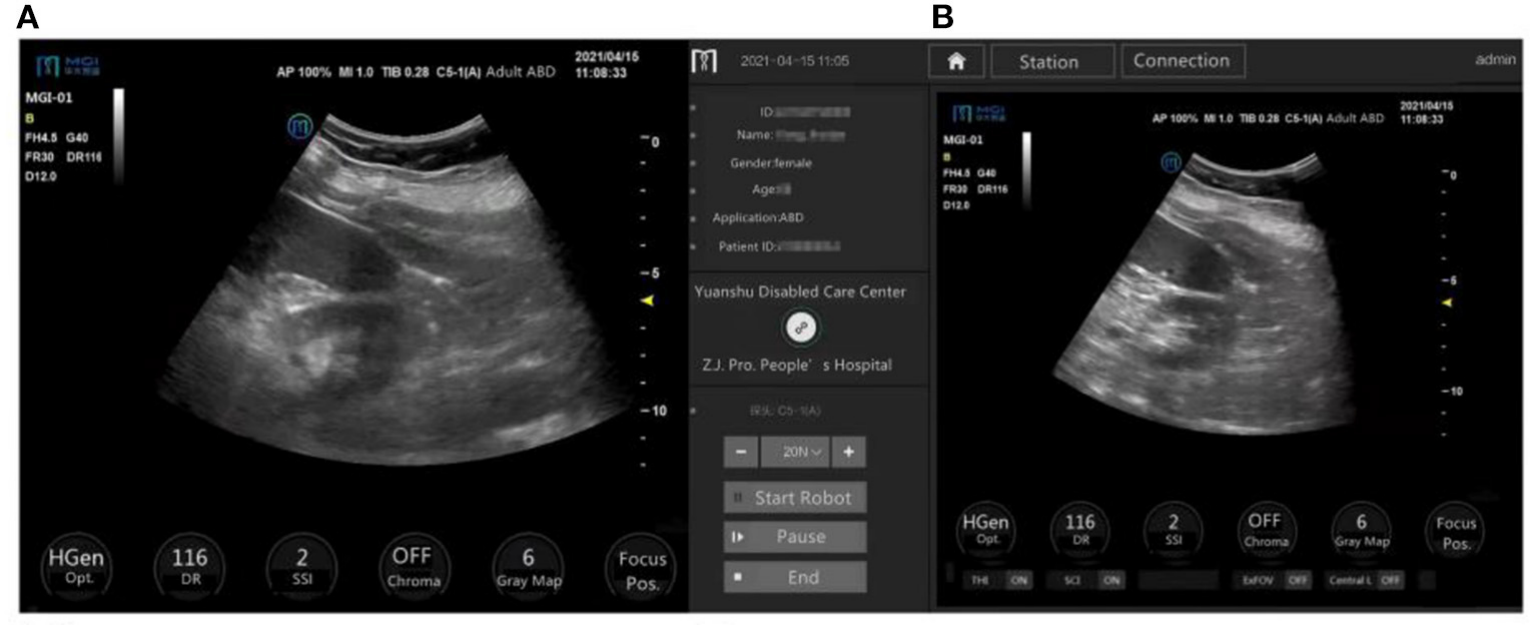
Ultrasound images on the patient side (original images) and doctor side (transmitted images) of the same organs in the same patient. The ultrasound image of the left kidney and the inferior pole of the spleen, displayed on the patient-side, scored 4.3 points on average (A), and on the doctor side scored 4.7 points on average (B) according to three independent experts.
